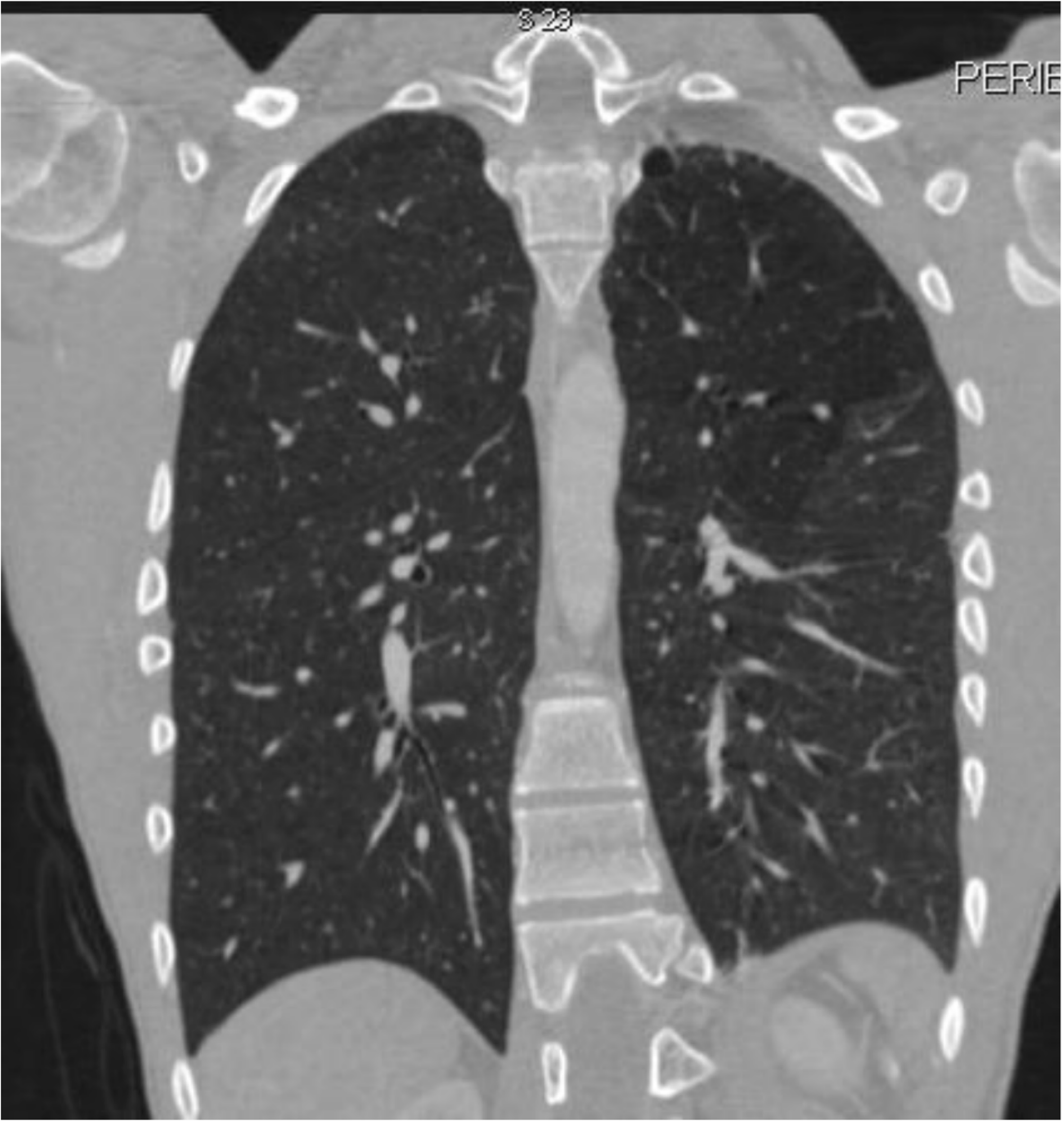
CT scan angiography of the same patient. Note the decreased vascularity of the left lung with hyperlucent upper lobe and presence of apical bulla. The left lung is smaller in comparison to the right.
